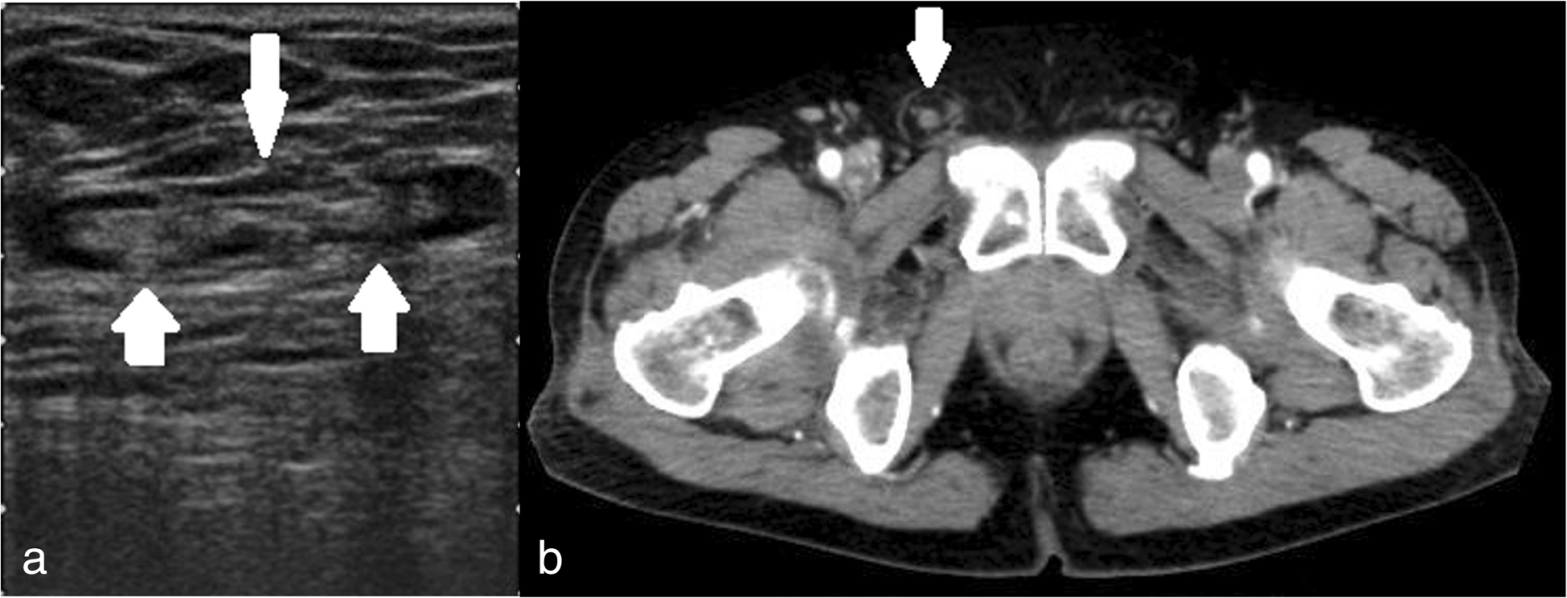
Preoperative imaging. a Ultrasonography showing non-inflamed appendix (arrow) inside the right inguinal canal. b Axial computed tomography (CT) scan showing non-inflamed appendix (arrow) in right inguinal hernia canal
